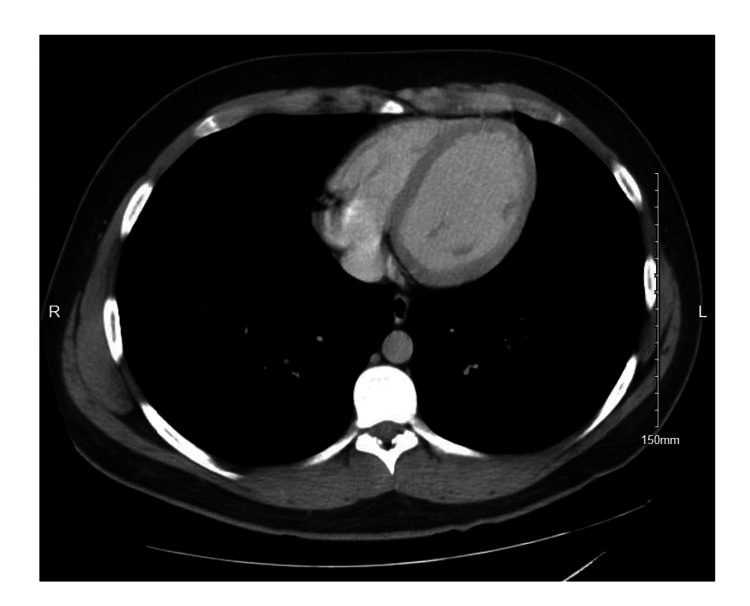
CT with contrast three weeks prior to admission CT abdomen/pelvis with contrast from three weeks prior to hospital admission demonstrating unremarkable exam.
